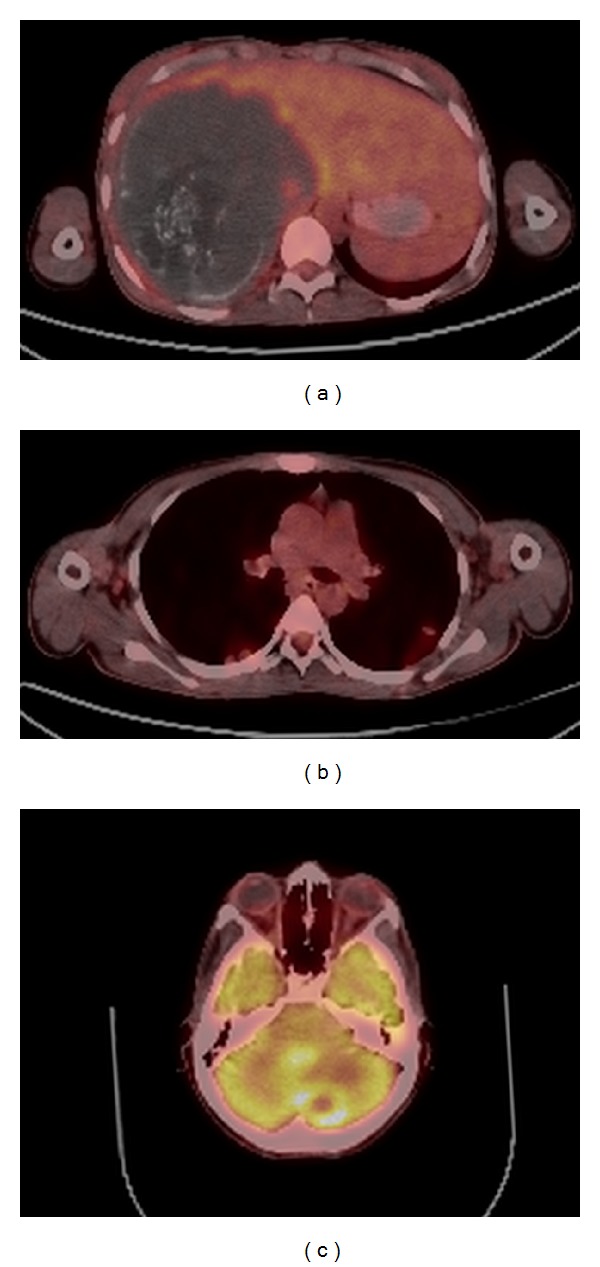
Whole-body PET-CT scan of the second case. AE lesion of the liver with only perilesional ^18^F-FDG uptake was seen on fused image (a). The evaluation of pulmonary nodules with PET-CT showed minimally increased metabolic activity (b). An axial slice from cerebellum revealed focal increased FDG uptake in the peripheral zone of the lesion (c).
